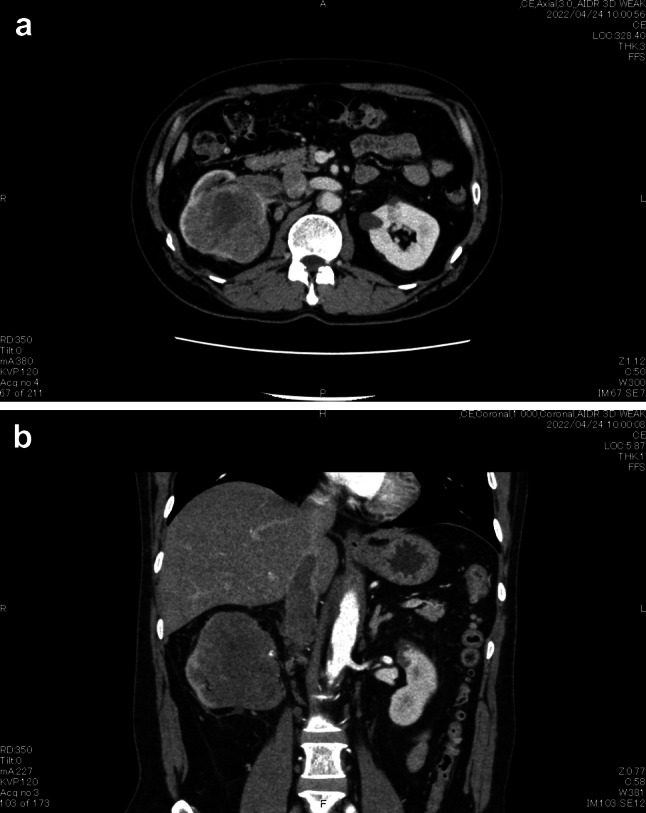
Images of the right renal tumor 3 months after presentation. a CT image showing a 78- × 76-mm right renal tumor. b Contrast-enhanced CT image showing a thrombus within the renal vein and a 77-mm-long thrombus in the inferior vena cava
